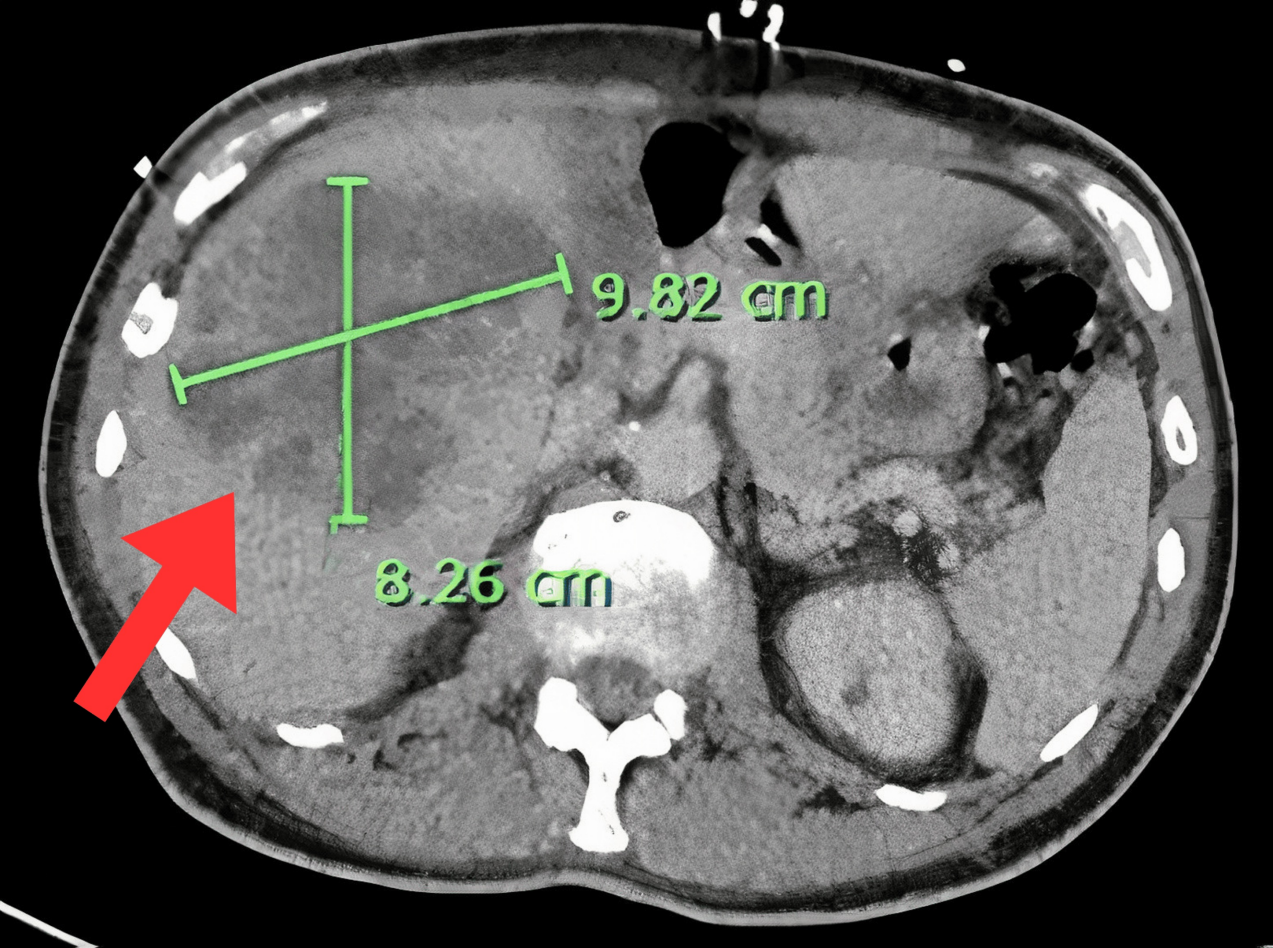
CT of the abdomen/pelvis with contrast done on admission showed a large multiloculated heterogeneous mass (red arrow) within the liver measuring 9.82 x 8.26 cm.
